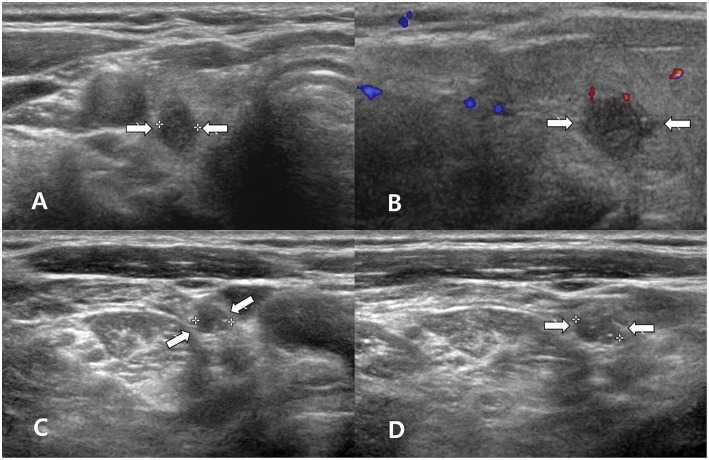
A 60~70 year-old woman with clear primary papillary thyroid carcinoma (PTC) on both ultrasonography (US) and cytology. Transverse gray-scale (A) and longitudinal color Doppler (B) sonography showed a solid thyroid nodule with spiculated margins and a non-parallel orientation in the right thyroid lobe (arrows, 0.5 × 0.8 × 0.9 cm; Korean Thyroid Imaging Reporting and Data system category 5). After US-guided fine-needle aspiration (FNA) of the right thyroid nodule, US-guided FNA for a suspicious lymph node in the right neck was performed; thyroglobulin measurement was also performed. Transverse (C) and longitudinal gray-scale (D) sonography showed a suspicious lymph node with increased echogenicity and diffuse vascularity in the right neck (arrows, 0.6 cm in length). Cytology for the right thyroid nodule indicated Bethesda category VI, but there was no malignancy in the lymph node by cytology and thyroglobulin measurement. After total thyroidectomy and right neck dissection, two PTCs (classic type) in the right thyroid lobe and multiple nodal metastasis from PTC in the right neck were confirmed.
